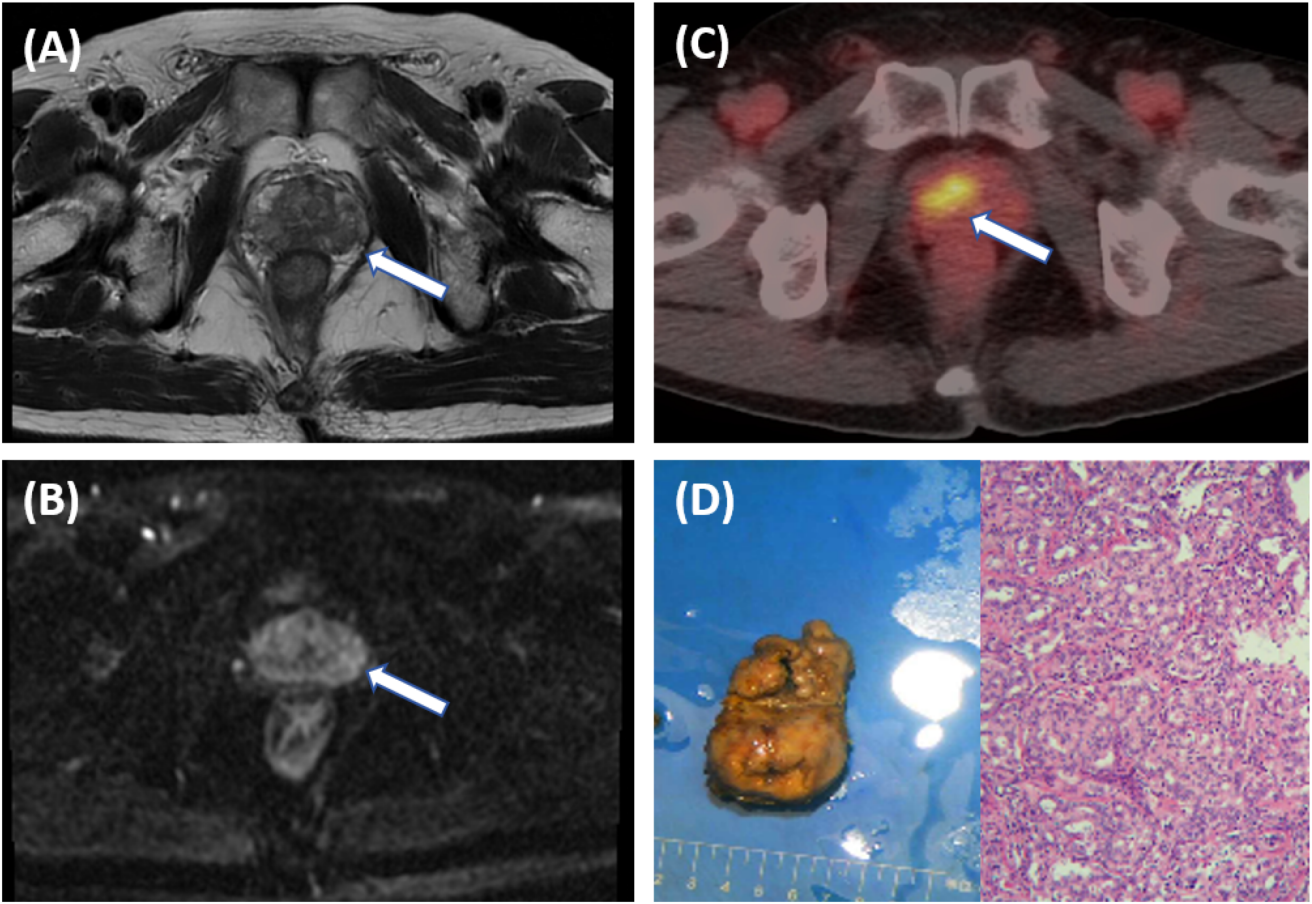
A 66-year-old male patient with an elevated PSA. Pelvic MRI shows multiple patchy T2 hypointense shadows in the bilateral peripheral zones of the prostate [(A), arrow], and slightly high signal on DWI [(B), arrow], with a PI-RADS score of 3, suggesting prostatitis. Further [18F]PSMA- 1007 PET/CT shows significantly increased PSMA expression on the right side of the middle region of the prostate [(C), arrow], with SUVmax of 13.4, suggesting prostate cancer. The postoperative pathological results indicate prostate adenocarcinoma with a Gleason score of 3 + 5 = 8 (D).
